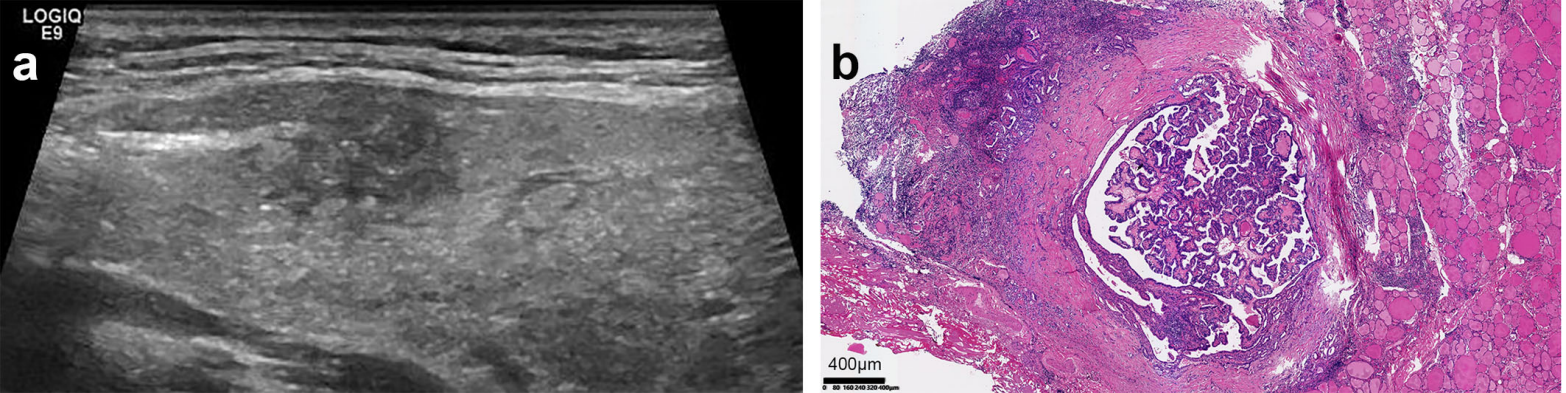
Ultrasound and pathological images of PTC with HT. (a) The parenchymal echo of the thyroid gland was thickened and less homogeneous. The node exhibited the following sonographic features: a discontinuous ventral dorsal membrane anteriorly, scattered punctate hyperechoic foci, heterogeneous internal echogenicity, irregular margins, and poorly defined borders. (b) Pathology result: (HE, x40)papillary thyroid carcinoma infiltrating the capsule, accompanied by Hashimoto’s disease changes in the surrounding thyroid.
